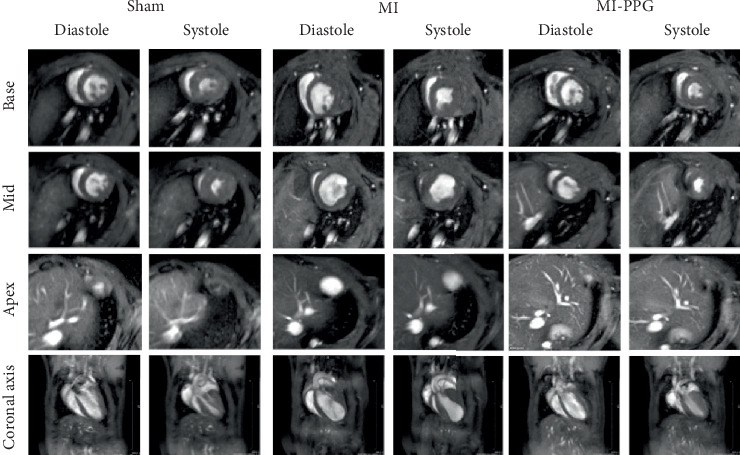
Cardiac MR imaging. Exemplary cardiac MR images 7 days after MI. Images show diastole and systole in axial planes from base to apex and coronal axis.
